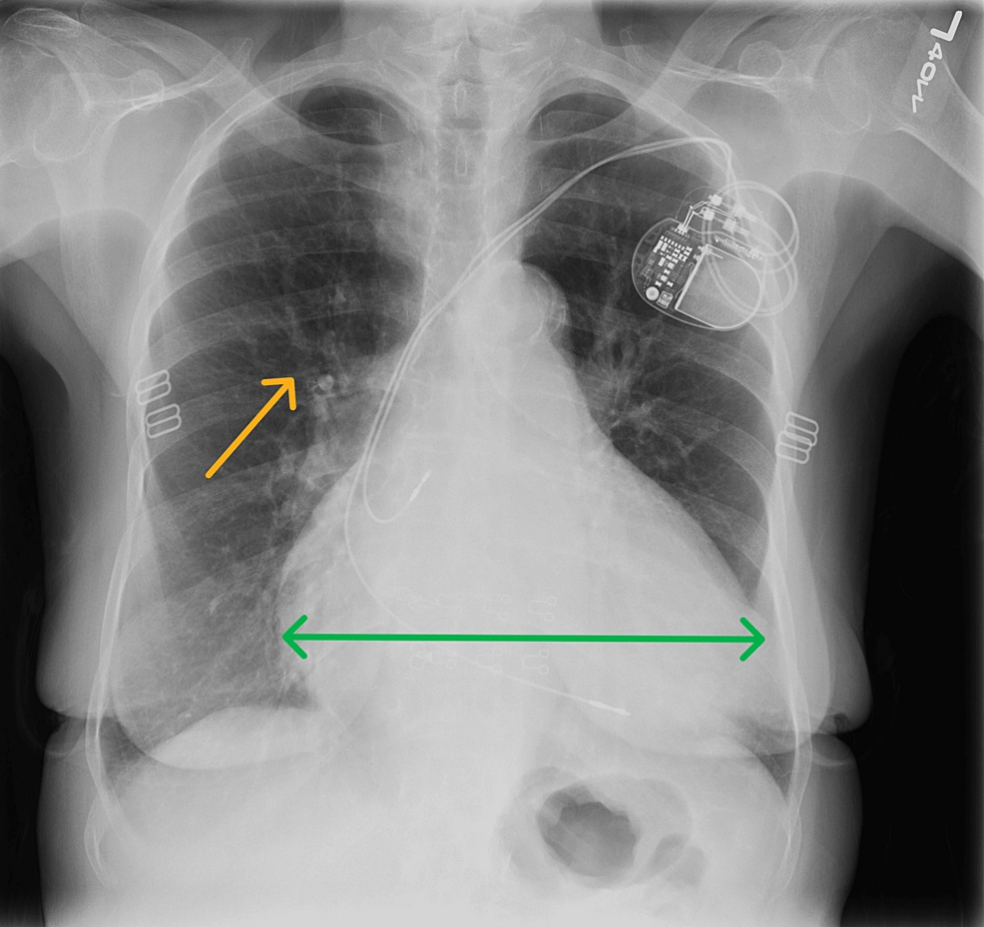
Chest X-Ray Suggestive of Volume Overload Green Arrow- Cardiomegaly; Yellow Arrow- Pulmonary Congestion
